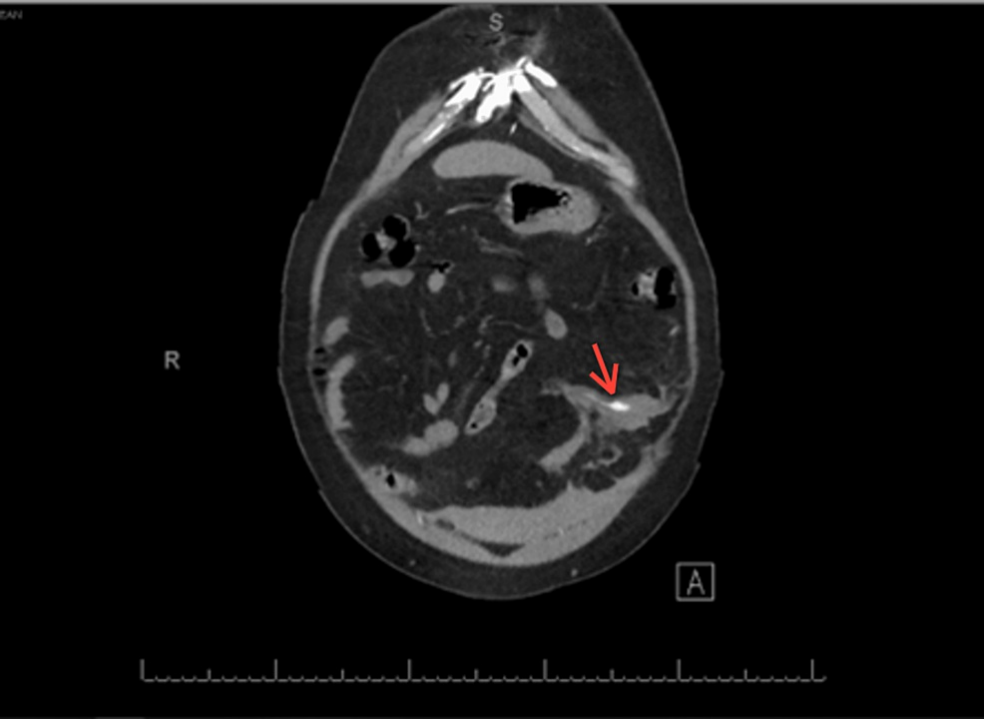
Enhanced CT scan axial view showing focal dilation of the omental artery.
